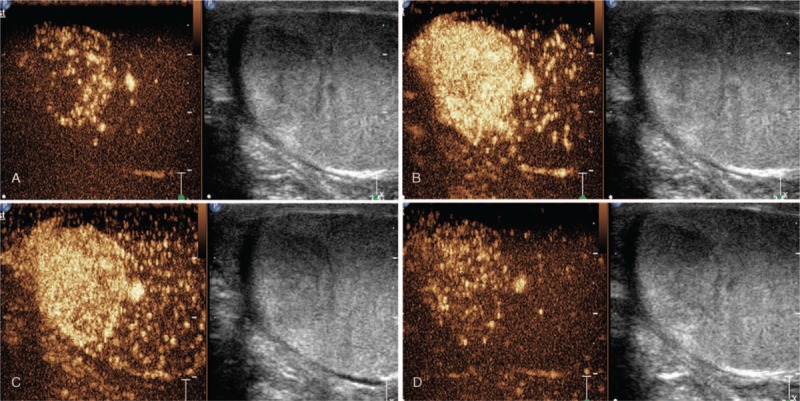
Longitudinal image of the testis. The left panel shows the CEUS mode, and the right shows the gray-scale mode. (A) CEUS image obtained 12 s after injection of microbubbles reveals a mass with peripheral enhancement. (B) The mass exhibits homogeneous enhancement at 22 s. (C) At 37 s, the contrast agent began to subside in a few areas. (D) The enhancement of microbubbles has diminished but is still more prominent than in the surrounding testicular area at 185 s. CEUS = contrast-enhanced ultrasonography.
